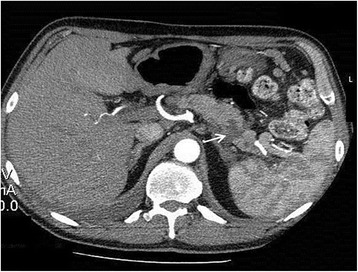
Post-contrast computed tomography scan of the upper abdomen reveals a small pseudocyst of 2cm at the pancreatic tail (arrow).
